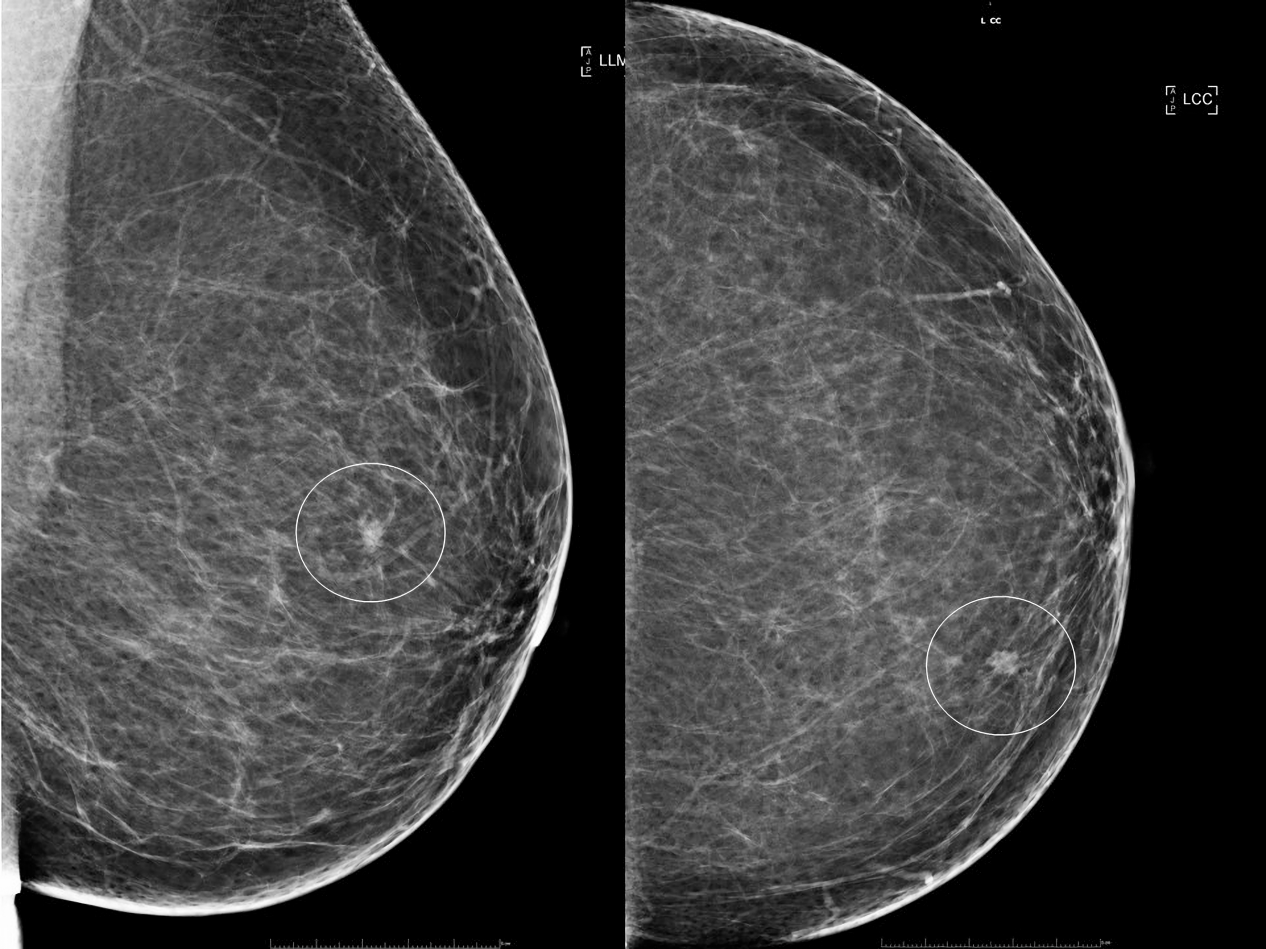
Plain x-ray mammograms demonstrating a small spiculated mass (circled) within the upper inner segment of the breast. Left image: Mediolateral oblique view. Right image: Craniocaudal view.
